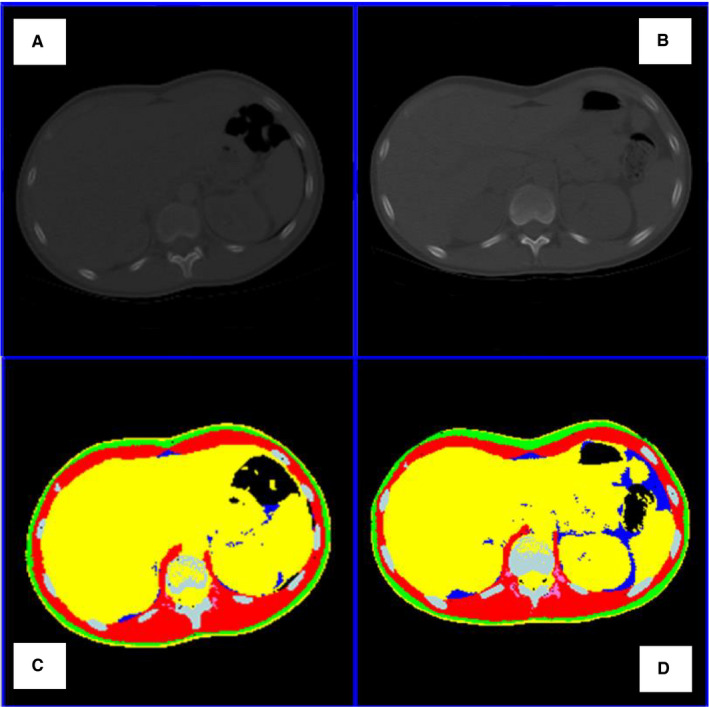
Single Slice Tissue Mass Quantification and Changes Over Time at the Level of T12‐L1 in an Adolescent Osteosarcoma Patient. A & B: Original chest CT scans at diagnosis (A) and following 2 cycles of neoadjuvant chemotherapy (B). C & D: Same images segmented, and each tissue tagged with a distinct color, allowing for tissue area quantification and comparison over time.
